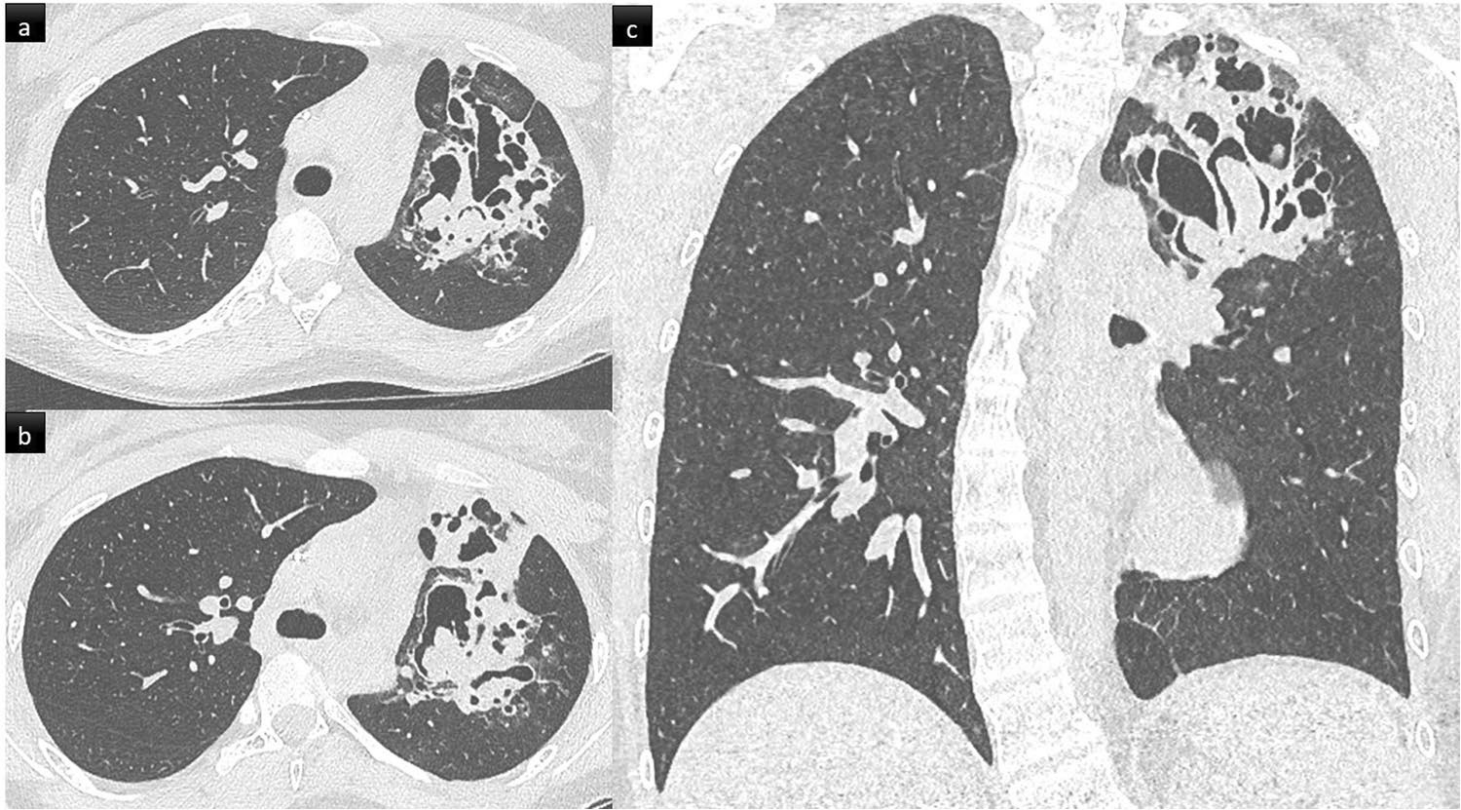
Chronic aspergillosis in a 32-year-old patient with autosomal dominant hyper IgE syndrome (HIES). Axial (a, b) and coronal mIP (c) CT scan images. Large cavities in the left upper lobe (a, c) with evidence of intra cavitary aspergillomas. Note the thoracic scoliosis, which is a frequent finding in HIES
